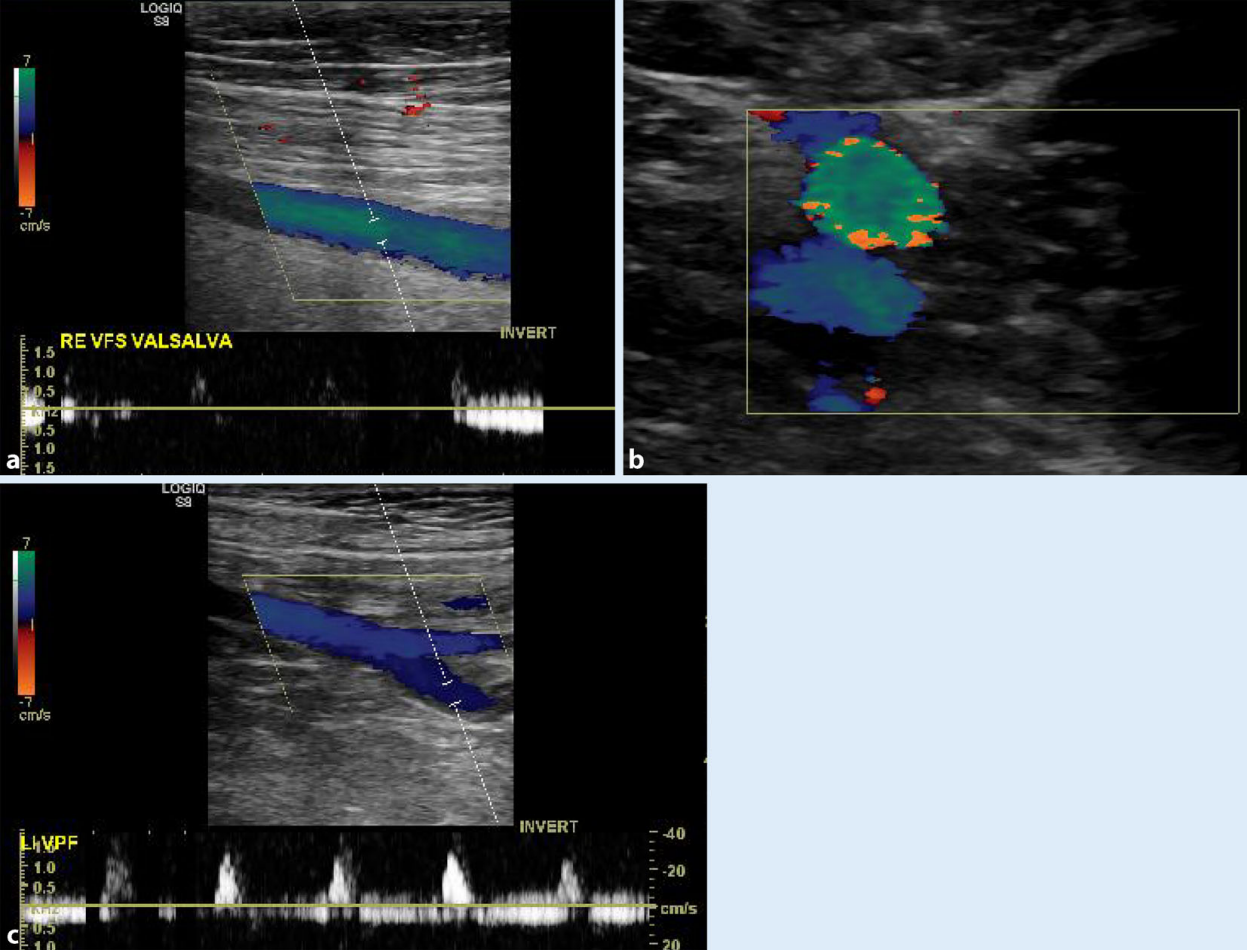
Duplex sonography showing a a normal and b a bifid superficial femoral vein and c junction of the deep femoral vein
